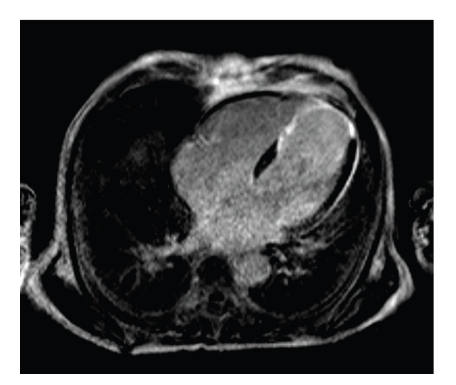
Four-chamber CE-MRI revealed an extensive transmural anterior AMI with septal involvement.
